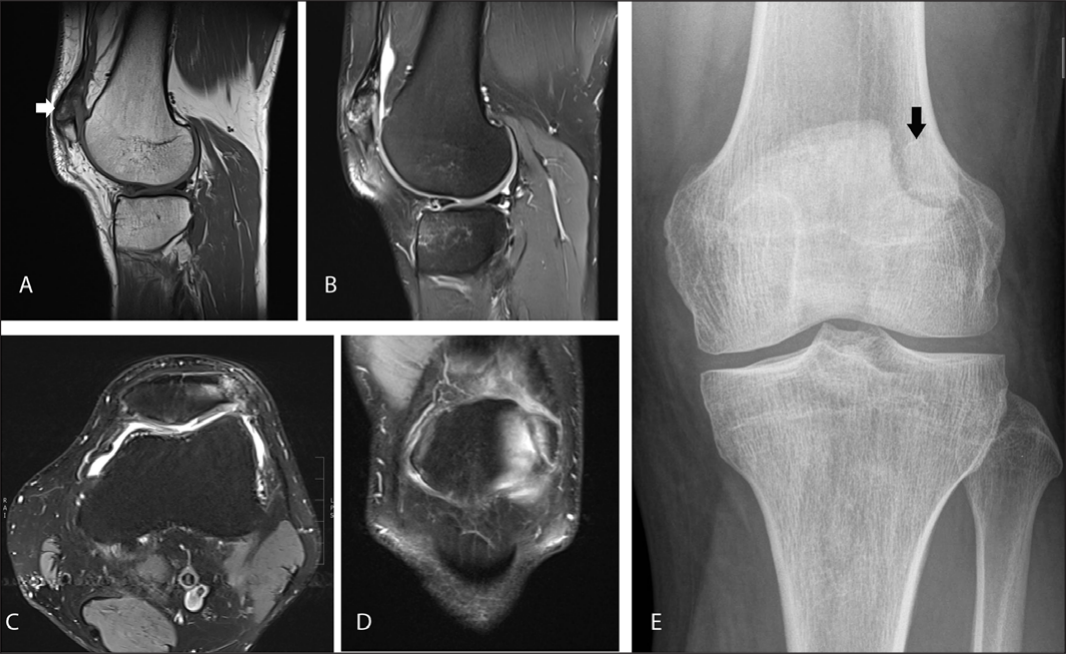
Symptomatic bipartite patella. Sagittal T1-WI (A) shows a separate bone fragment at the superolateral aspect of the patella (arrow). Sagittal (B), axial (C) and coronal (D) FS T2-WI reveal bone marrow edema within the accessory fragment and the adjacent superolateral aspect of the patella. Plain radiography (E) confirms a sclerotic delineated accessory fragment at the superolateral aspect of the patella (arrow).
